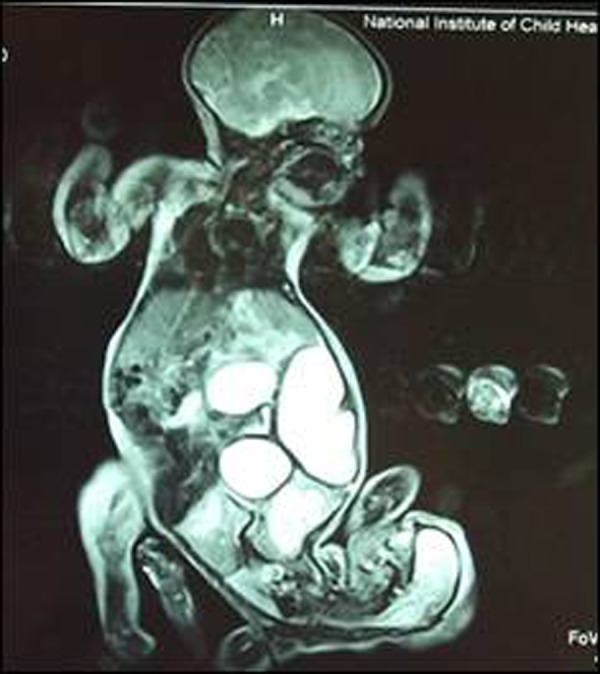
Figure 5: MRI of conjoined twins
